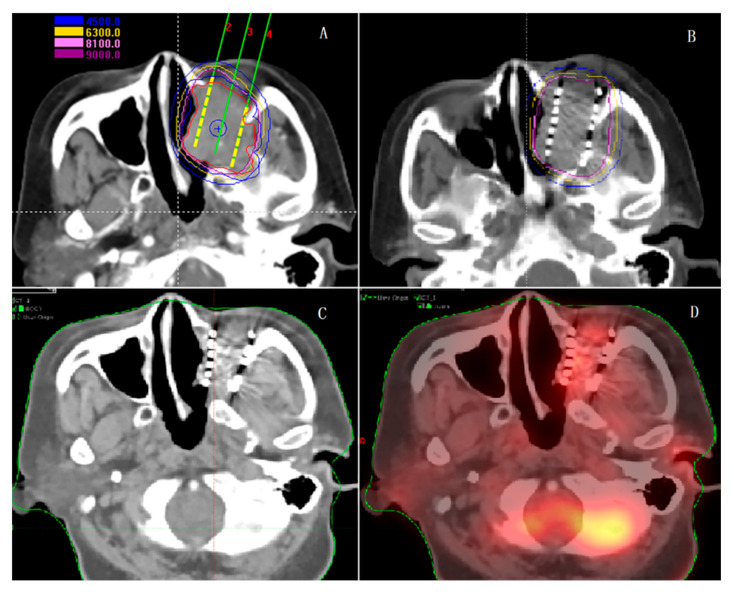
Clinical stereotactic ablative brachytherapy (SABT) practice for head and neck cancers. (A): Preoperative planning for a head and neck cancer; (B): Intraoperative replan and seed implantation; (C,D): the cancer is shrinking 3 months after seed implantation with low in-taking of fluorinated deoxy-glucose on the PET/CT image (SUV < 2.5).
